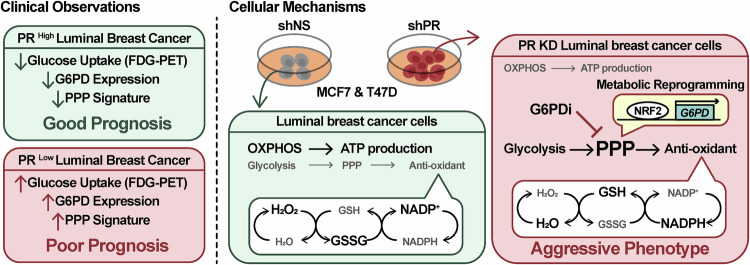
A proposed model for PR-loss-driven metabolic reprogramming and therapeutic targeting in luminal breast cancer. This model contrasts the clinical observations in patients with the underlying cellular mechanisms. Left (Clinical Observations): PR expression status defines distinct clinical phenotypes. Low PR expression is associated with an aggressive profile characterized by a cascade of observable features: increased Glucose Uptake (FDG-PET), elevated G6PD Expression, and an activated PPP Signature. This combination of metabolic and molecular markers is ultimately linked to a Poor Prognosis. Conversely, high PR expression is characteristic of a less aggressive phenotype with reduced levels of these markers, leading to a Good Prognosis. Right (Cellular Mechanisms): Mechanistically, loss of PR leads to NRF2 activation, which directly binds to the G6PD locus to induce its transcription. The resulting G6PD upregulation enhances PPP flux, increasing the NADPH/NADP⁺ and GSH/GSSG ratios to strengthen antioxidant capacity. This metabolic rewiring supports tumor aggressiveness and establishes a critical dependence on G6PD, which can be therapeutically exploited using G6PD inhibitors to suppress tumor growth.
